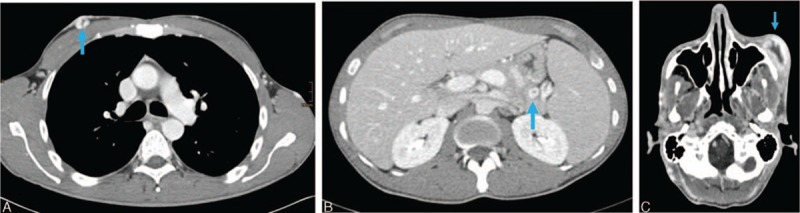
Contrast-enhanced CT of the patient with bacillary angiomatosis showing multiple abscesses (blue arrows). Axial CT images. (A) Chest: subcutaneous abscess in contact with the right pectoralis muscle. (B) Abdomen: abscess in the tail of the pancreas. (C) Head: massive abscess at the left zygomatic arch with bone erosion. CT = computed tomography.
